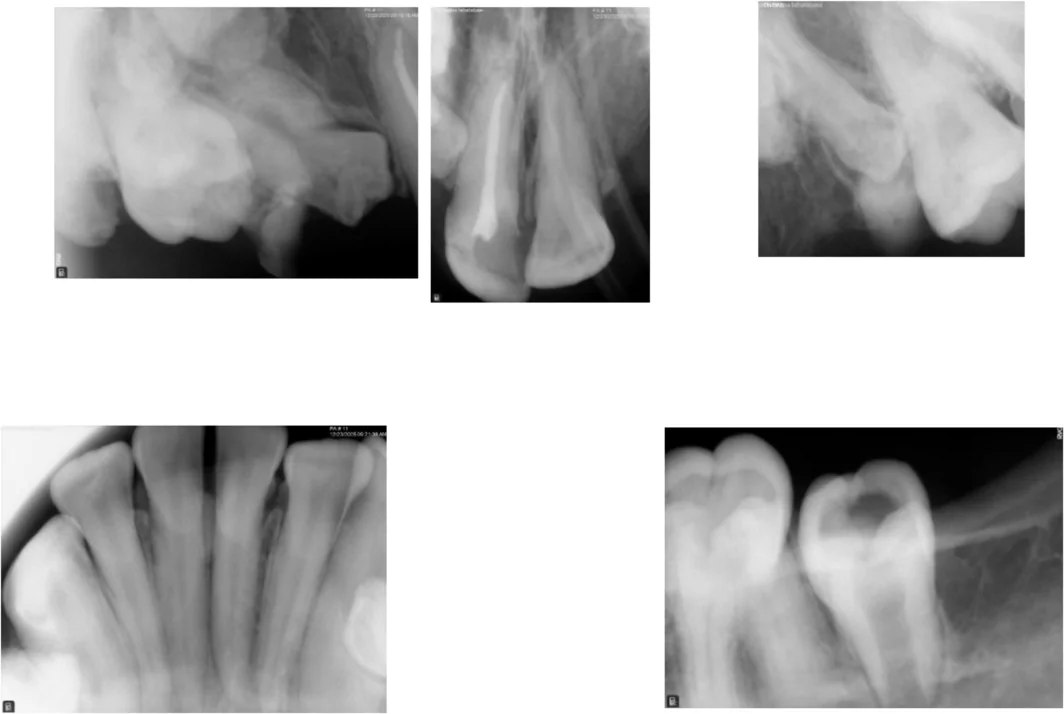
Preoperative intraoral periapical radiographs obtained in 2025 (1404 SH).
